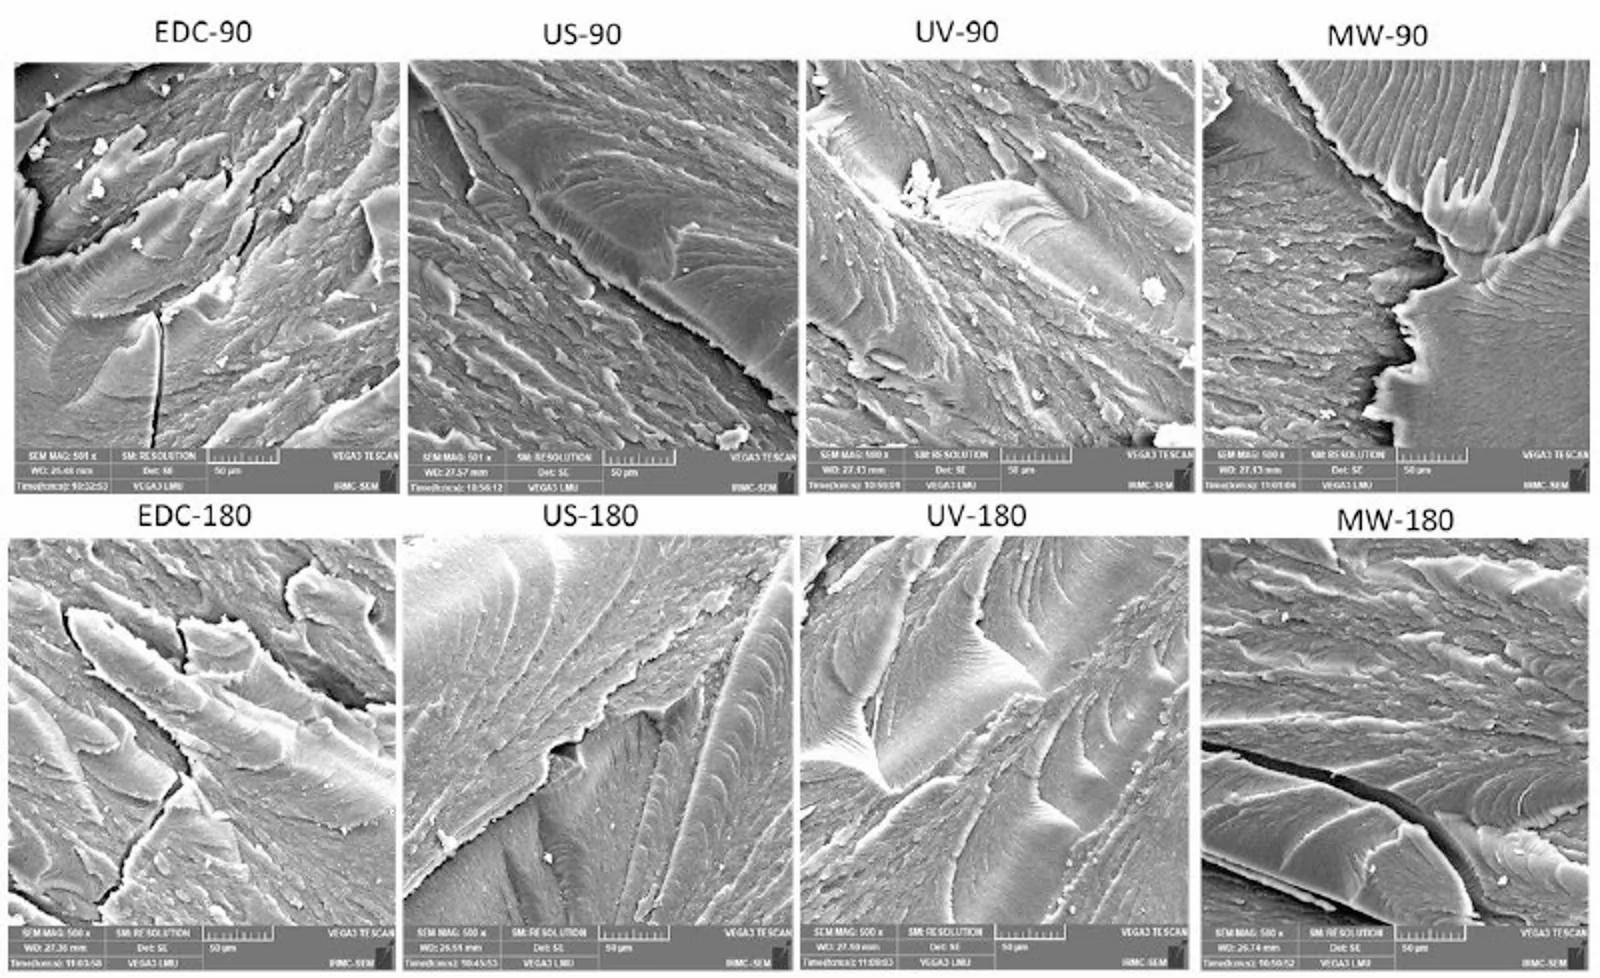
Show the SEM images of disinfection groups for DentaBase under x500 (ESC) Effervescent denture cleanser tablets (US), Ultrasonic, (UV) Ultraviolet, (MW) Microwave
